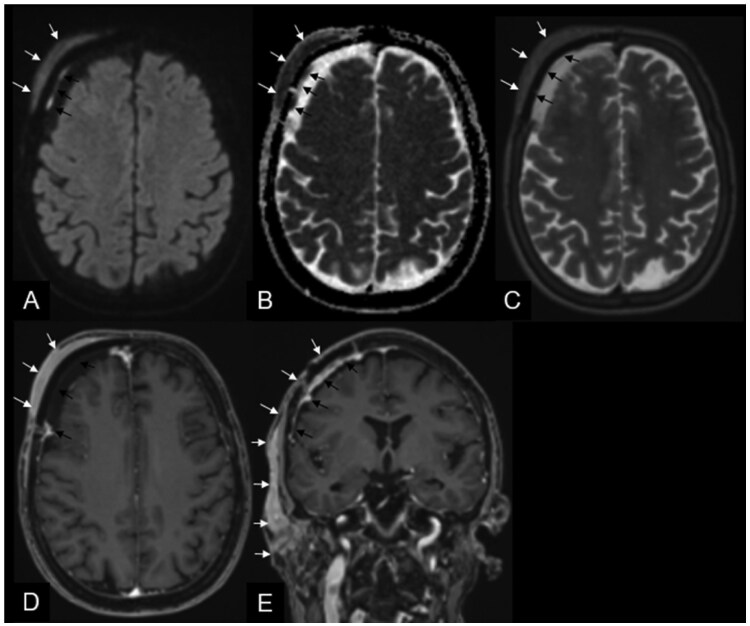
Follow-up magnetic resonance imaging (MRI) 3 months after gross total resection. The brain MRI revealed a soft tissue mass at precisely the same subgaleal location as the primary tumour (white arrows A–E), with new invasion into the adjacent subcutaneous fat and skin. The recurrent tumour had expanded into adjacent anatomical regions, involving the right frontotemporal region, involving the parotid space, directly infiltrating both the superficial and deep lobes of the right parotid gland, and extending to the posterior right masticator space. The MRI characteristics were similar to those of the primary tumour, raising the possibility of intraoperative tumour seeding. The black arrows in A–E point to the patient’s specific implant with primary reactive granulation tissue in the course of the cranioplasty.
